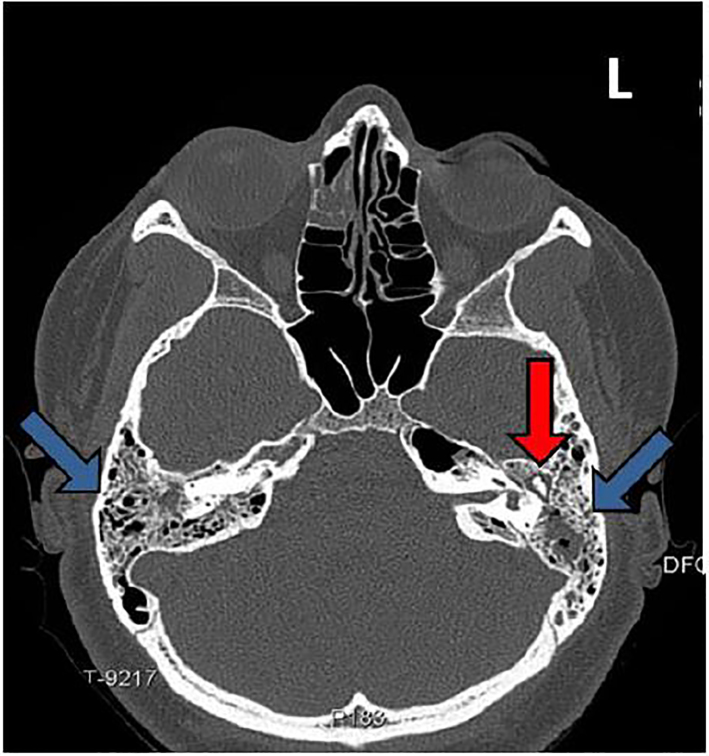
Axial computed tomography of the mastoid shows near complete opacifications of bilateral mastoid air cells with coalescence and erosions (blue arrows). Fluid is seen surrounding the ossicles of the left middle ear (red arrow).
